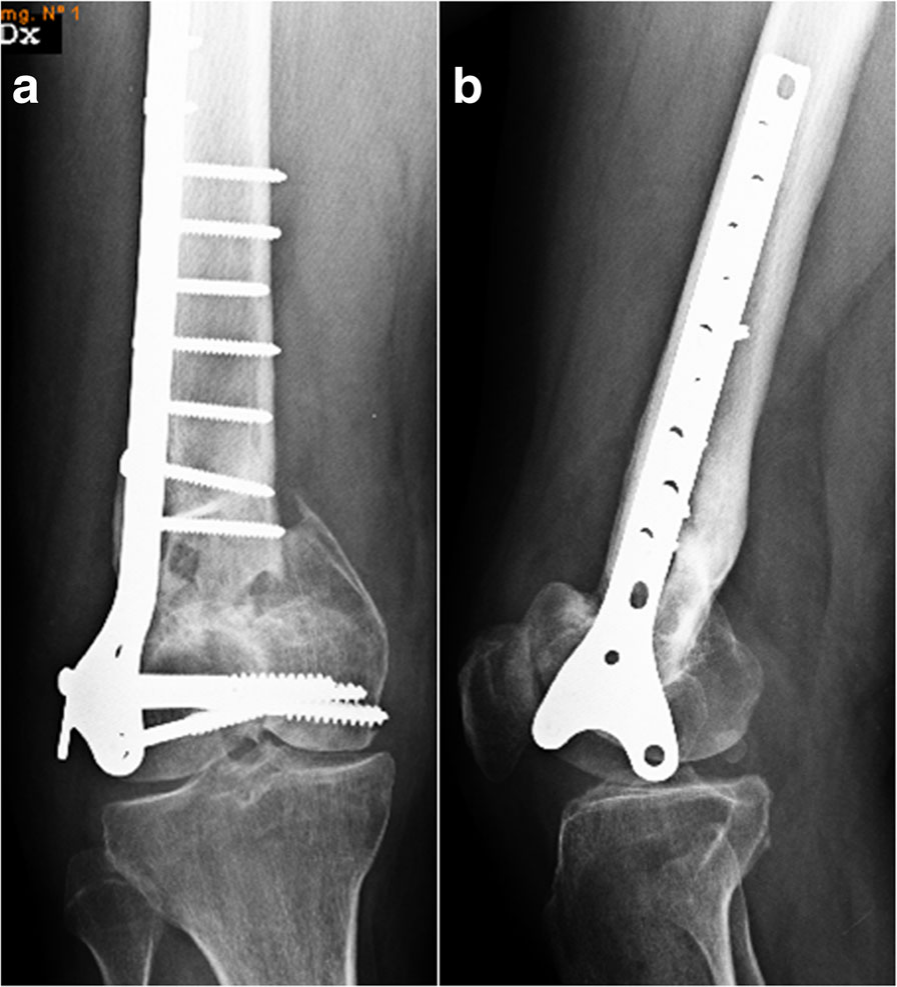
a Anteroposterior radiograph pre-operative of hardware removal; b Lateral radiograph pre-operative of hardware removal
